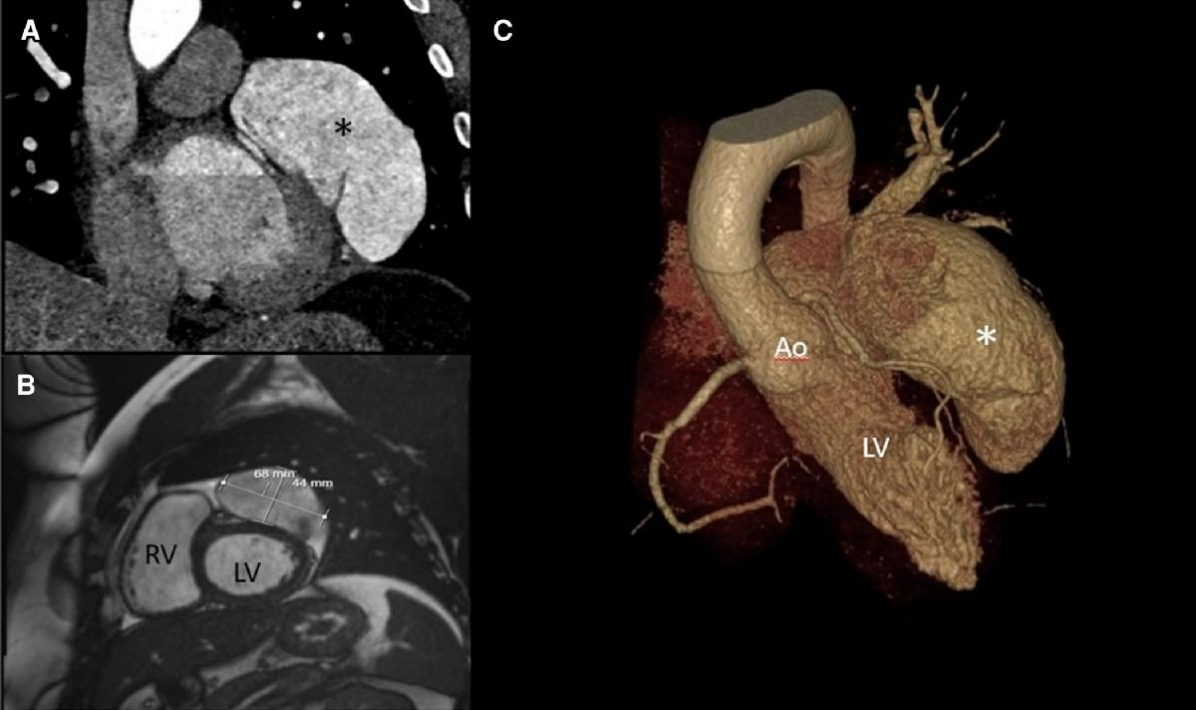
(*A*) CT scan of the heart in coronal plane depicting the LAAA (asterisk) in contact with the LV lateral wall. (*B*) CMR steady-state free precession image in diastole showing the LAAA exerting compression on the anterior and anterolateral LV walls. (*C*) CT 3D reconstruction showing the LAAA (white asterisk) in close approximation to the LV myocardium, the left anterior descending artery, and left superior pulmonary vein.
